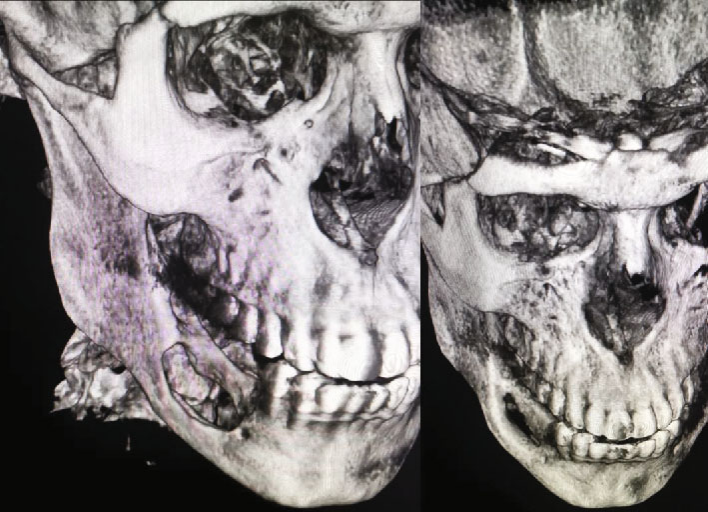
Preoperative and one-year postoperative 3D CT scan.
